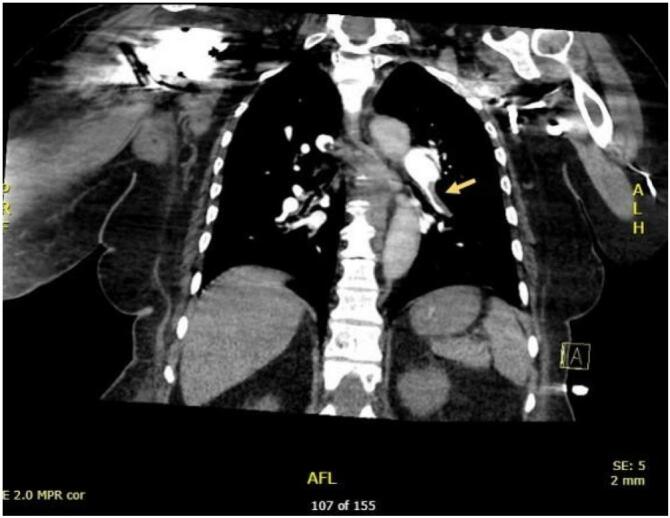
Spiral computed tomography showing left lower lobe filling defect respectively suggestive of pulmonary embolism.
